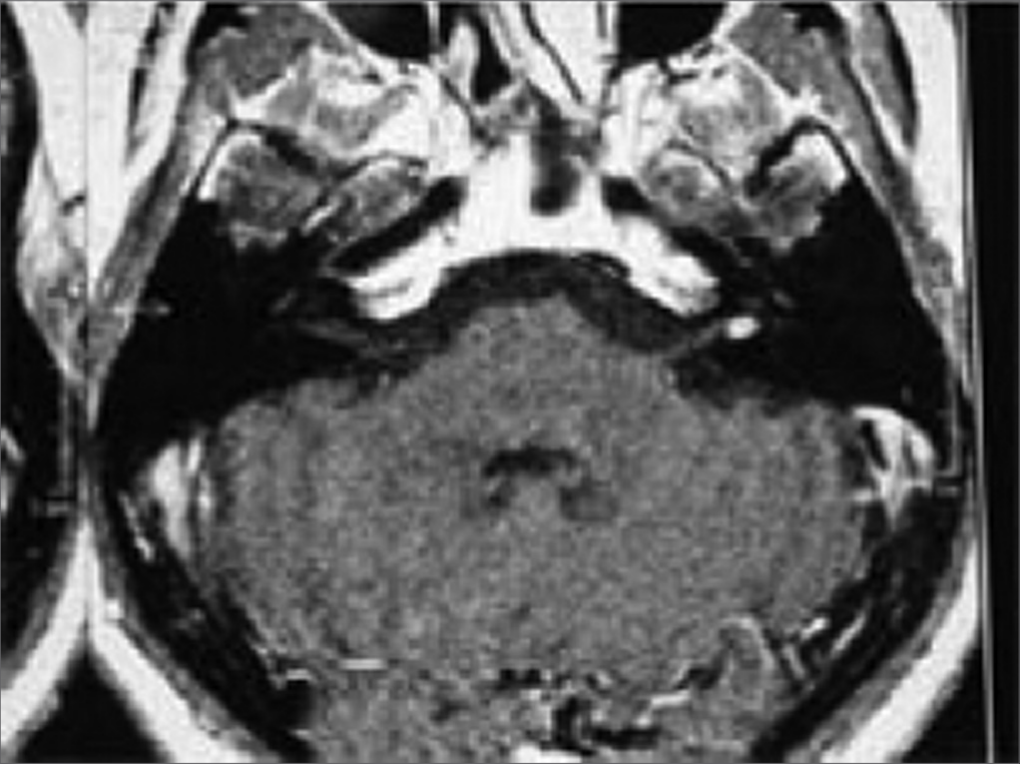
MRI with Vestibular Schwannoma, axial view.
